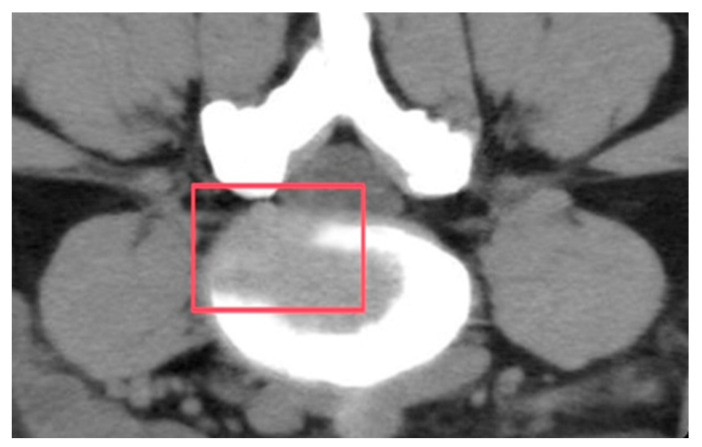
A CT scan image of the degenerated IVD (boxed region: degenerated disc).
